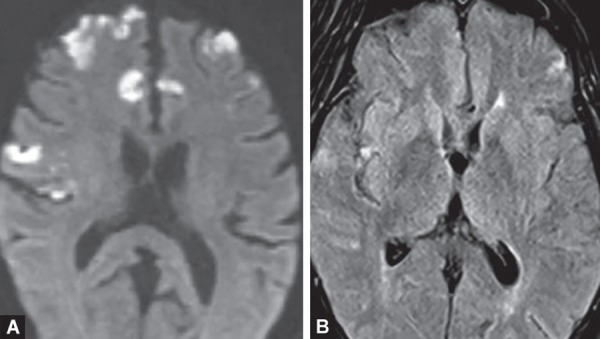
DWI sequence is more sensitive in detecting acute early age embolic infarcts which are not very well appreciated on FLAIR sequence
